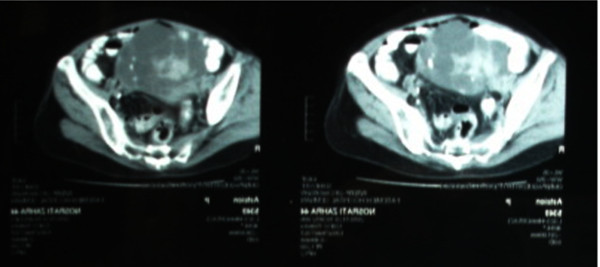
Pelvic CT scan of the patient showing the mass in the pelvic cavity.
